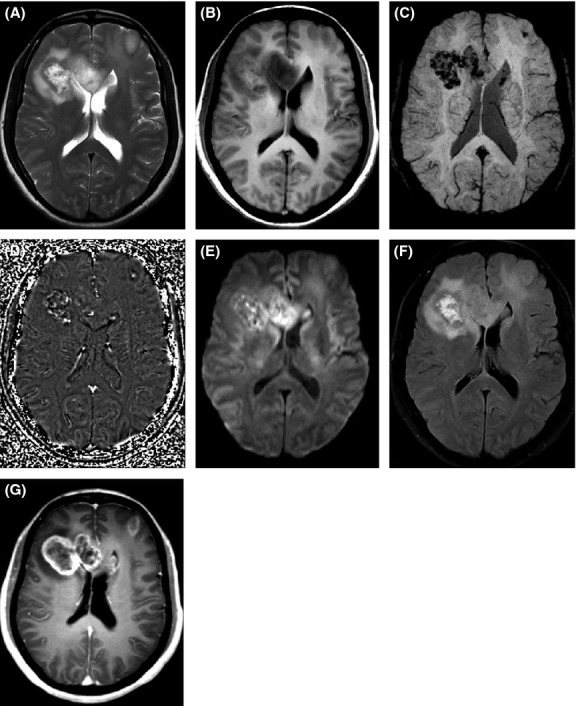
A 45-year-old female with glioblastoma (WHO grade IV). Three lesions were located in bilateral frontal lobe and genu corpus callosum on T2WI (A) and T1WI (B). In the lesion located in the right frontal lobe, the 2 dot-like low signals were found on T2WI. However, on SWI-MinIP (C), more hemorrhagic signals were found in lesions, which showed high signals on phase image (D), and a vessel was found in the lesion located in genu corpus callosum. The lesions showed hyperintense signal both on DWI (E) and FLAIR (F), and demonstrated ring, intense and inhomogeneous enhancement on contrast-enhanced T1-weighted image (G).
